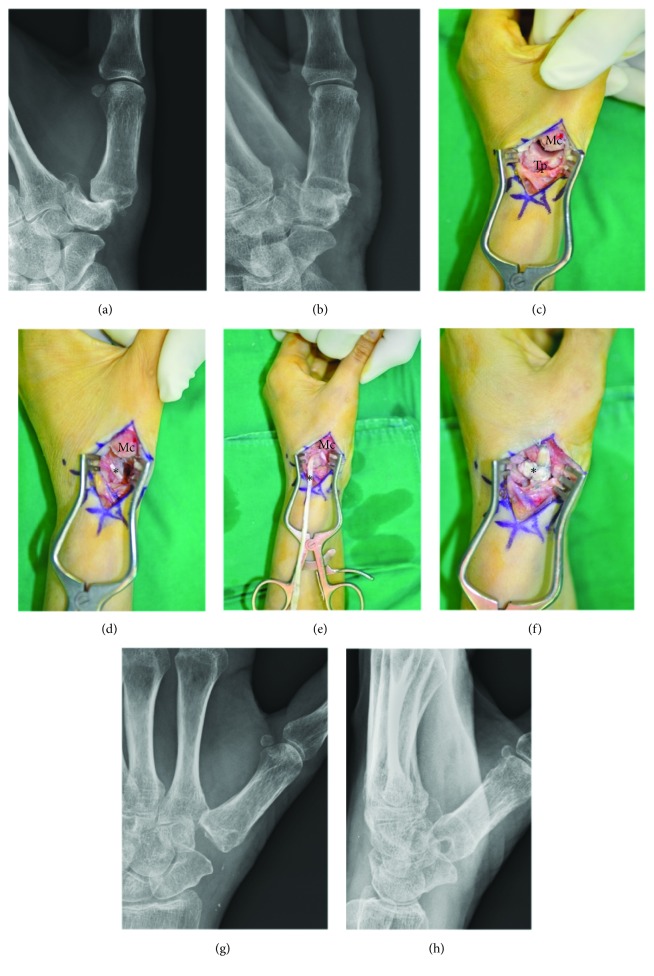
The ligament reconstruction and tendon interposition (LRTI) surgical procedure. (a, b) Posteroanterior and lateral radiographs of a 52-year-old female with Eaton-Littler stage III trapeziometacarpal (TMC) osteoarthritis. (c) After opening the dorsal capsule of the TMC joint, the base of the first metacarpal bone and trapezium were exposed. (d) The base of the insertion of the flexor carpi radialis (FCR) tendon of the second metacarpal bone was noted (*∗*) after excision of the trapezium. Care was taken to avoid FCR tendon injury. (e) The distally based radial half of the FCR tendon was harvested from its musculotendinous junction and retrieved distally to its insertion. (f) The retrieved tendon was delivered through the bone tunnel, and then the FCR remnant was weaved along its length using the anchovy procedure and interposed in the trapezium space and anchored deeply to the joint capsule. (g, h) Posteroanterior and lateral postoperative radiographs. Mc, metacarpal bone; Tp, trapezium.
